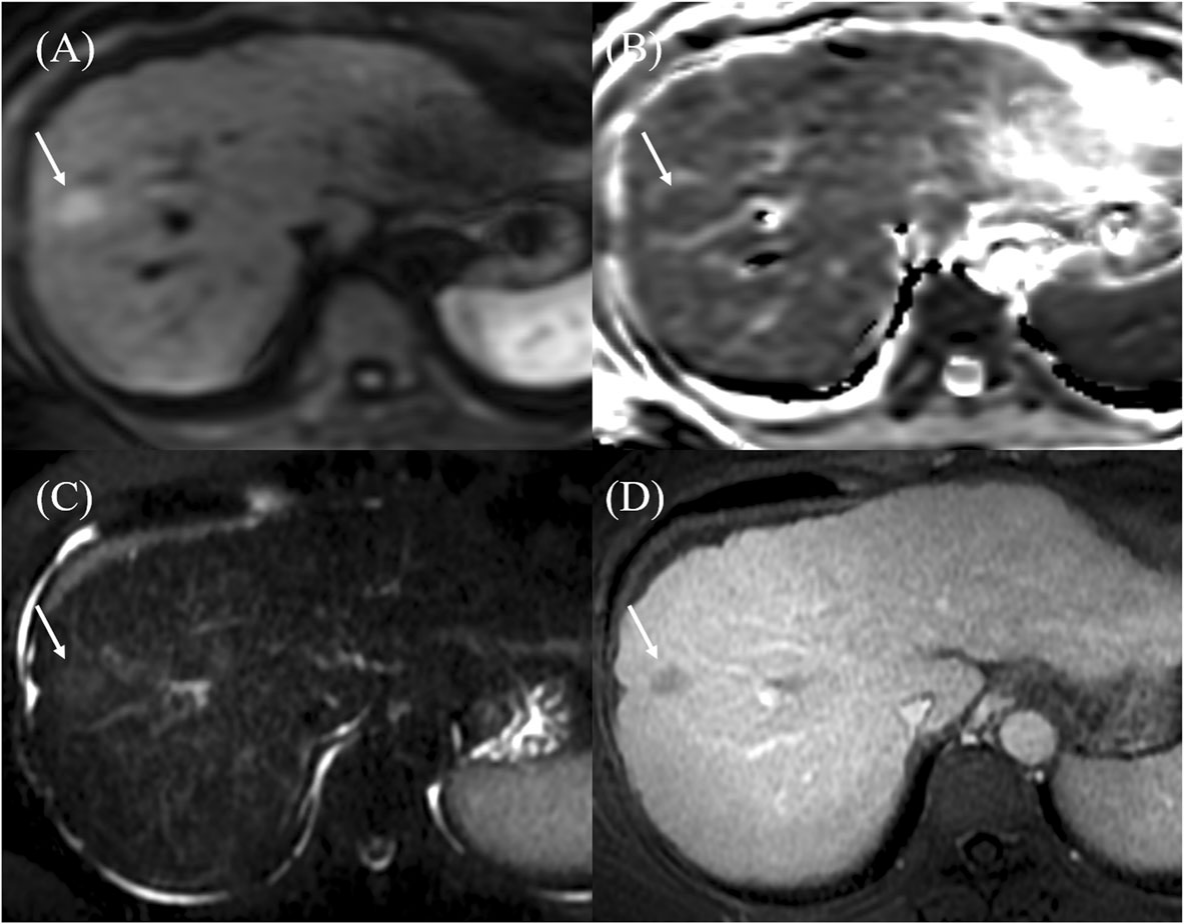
MR imaging in a 60-year-old woman. Pathologically confirmed grade 2 HCC. The tumor is hyperintense to surrounding liver parenchyma on a DWI (b = 400 s/mm^2^), slightly hypointense on b ADC map, and mild hyperintense on c T2-weighted imaging. d Postcontrast T1-weighted image shows minimal enhancement. ADC_min_ of tumor was 1.11 × 10^− 3^ mm^2^/s
